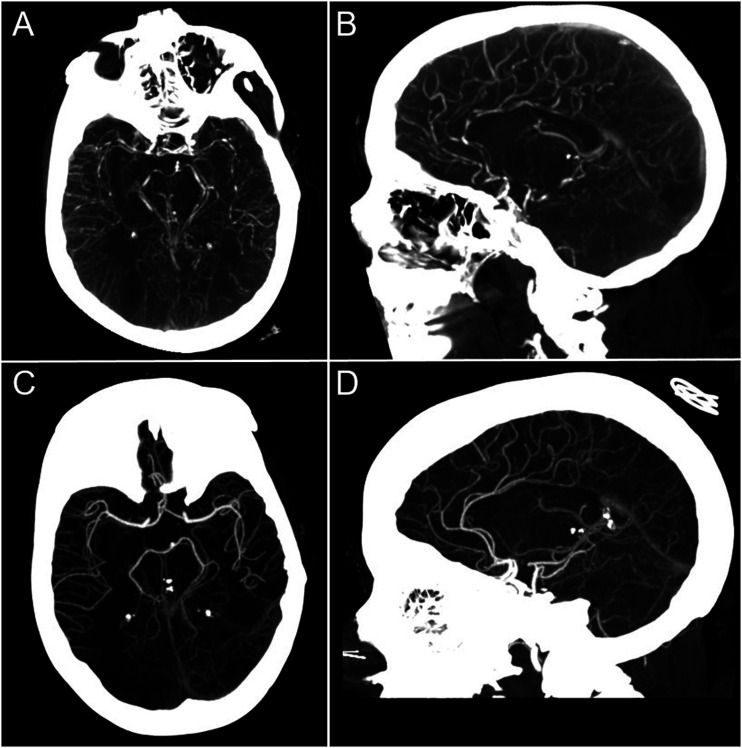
CTA showing multiple areas of segmental stenosis in the anterior and posterior circulations (A and B), near-complete resolution of the multifocal segmental stenoses seen on the original study (C and D).
